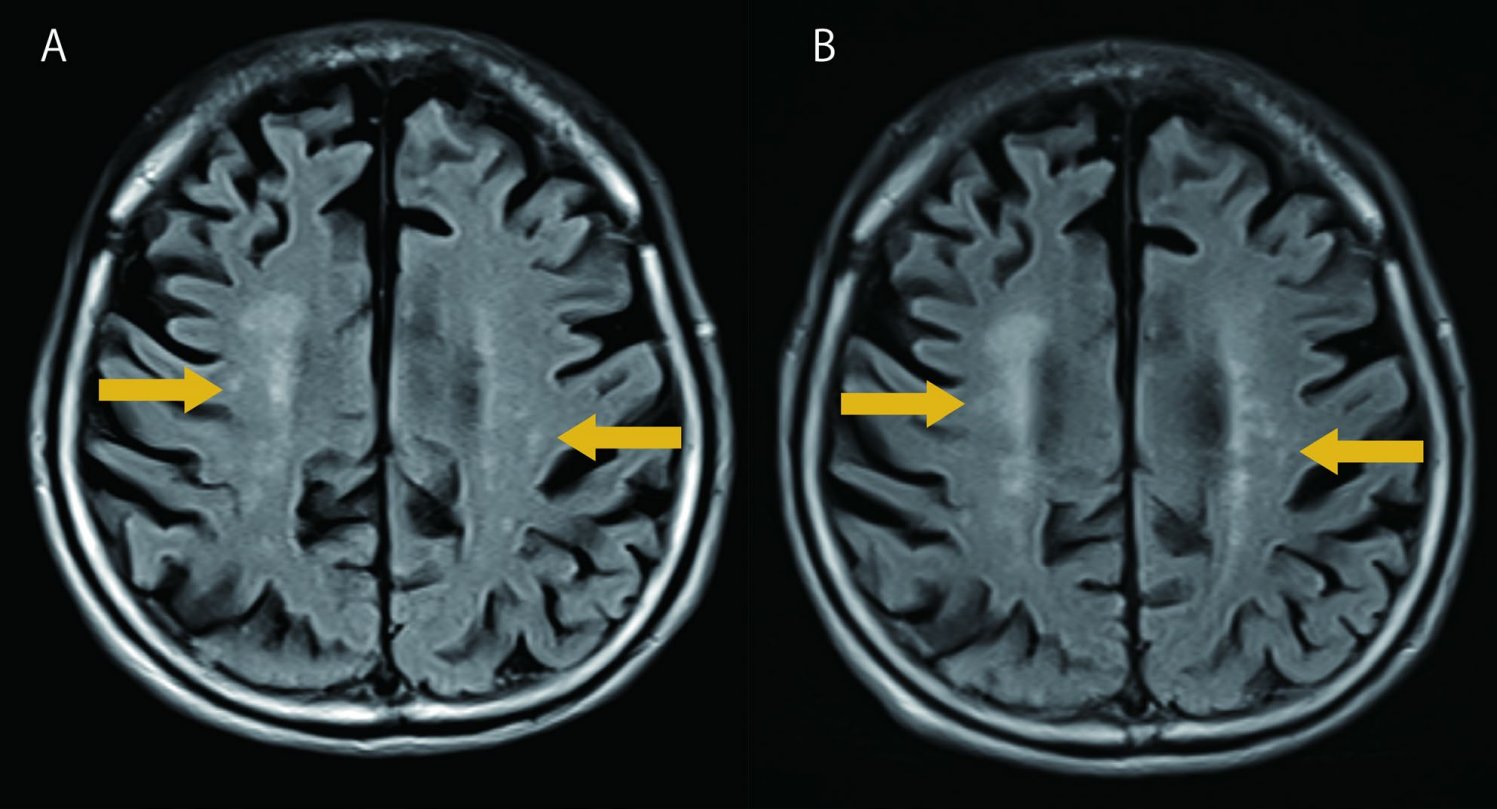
Serial FLAIR imaging demonstrating imaging-clinical dissociation in cerebral fat embolism. A Baseline FLAIR images obtained 7 days after symptom onset show multiple hyperintense lesions in the bilateral cerebral white matter, corpus callosum, and cerebellum. B Follow-up FLAIR images at 2 months show minimal interval change with persistent structural abnormalities in the same regions
